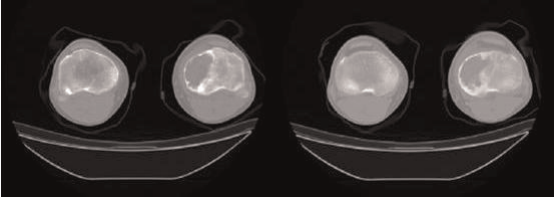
CT examination (sequential images at the level of the lesion) reveals slight bone expansion, cortical thinning and endosteal scalloping, indistinct margins and calcifications inside the lesion.
